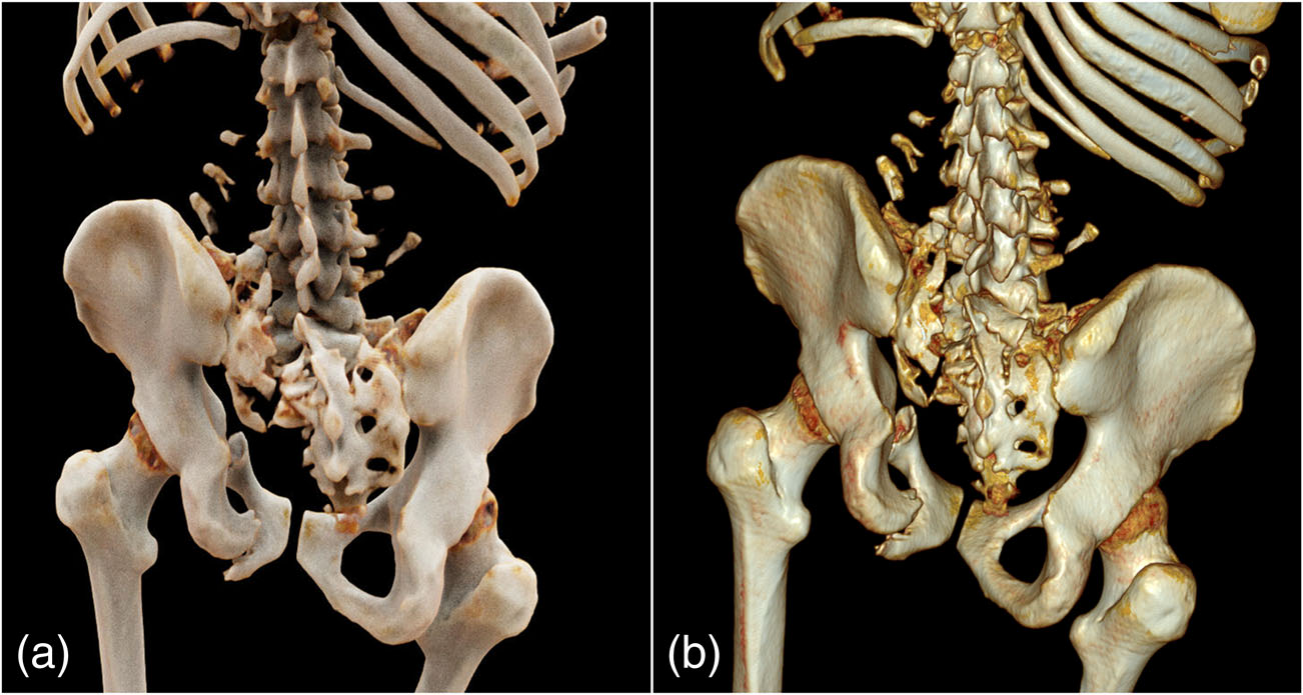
Non-enhanced 3-D CT images of multiple traumatic fractures of the spine and pelvis. Both CR (a) and VR (b) demonstrate multiple fractures of the ribs, lumbal transverse processes, sacrum, and pubic bones
